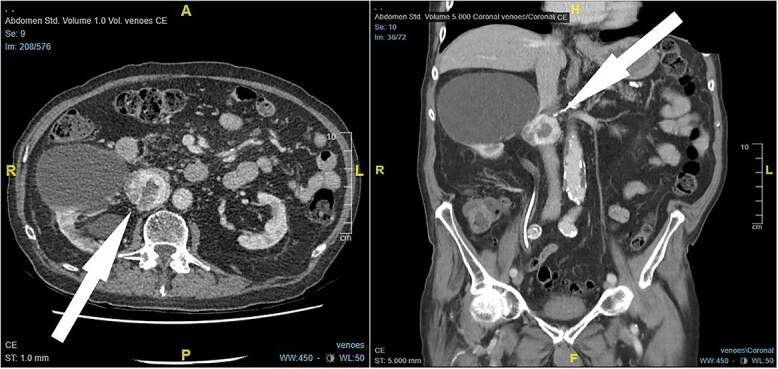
CT scan findings, 4.5 × 4 × 4 cm right retroperitoneal mass posterior to the inferior vena cava, with compression on the inferior vena cava and the right renal artery (arrow).
